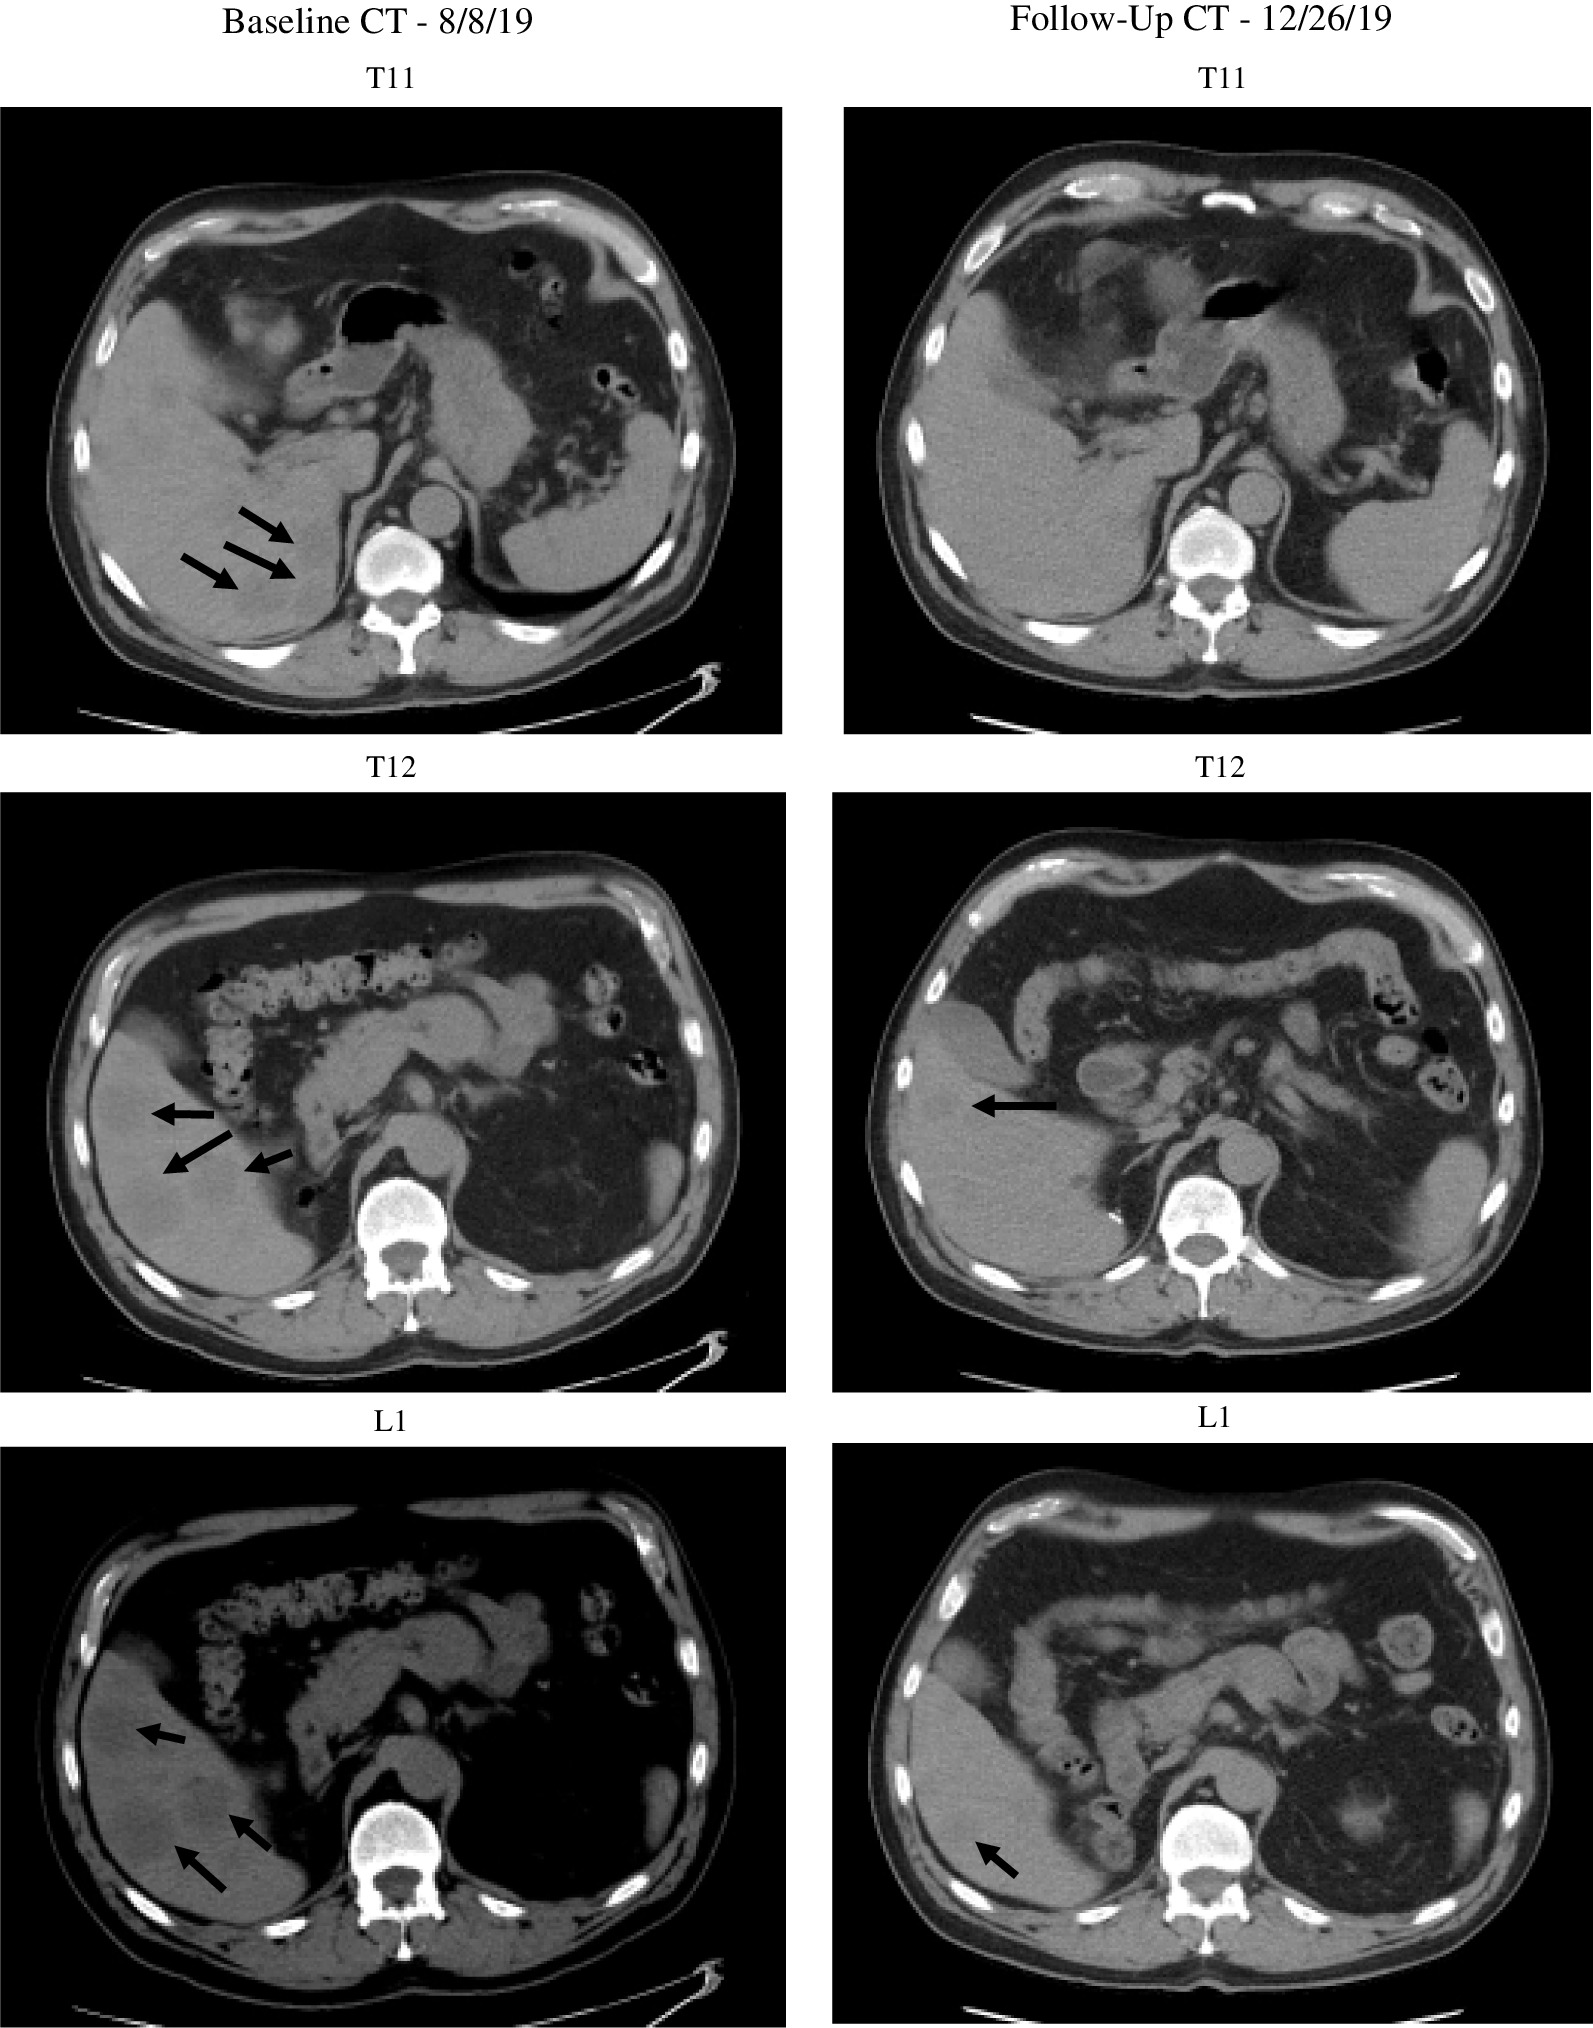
Axial contrast enhanced computed tomography scan of the abdomen and pelvis. Interval decreases in size of liver metastases with reference measurements are as follows: T11: 1.6 × 1.9 cm metastasis of liver towards the dome compared to baseline of 3.7 × 3.4 cm. T12: 1.6 × 2.2 cm metastasis towards the dome more centrally compared to baseline of 3.3 × 3.5 cm. L1: 2.1 × 2.1 cm metastasis inferior right hepatic lobe compared to baseline of 3.2 × 3.9 cm
